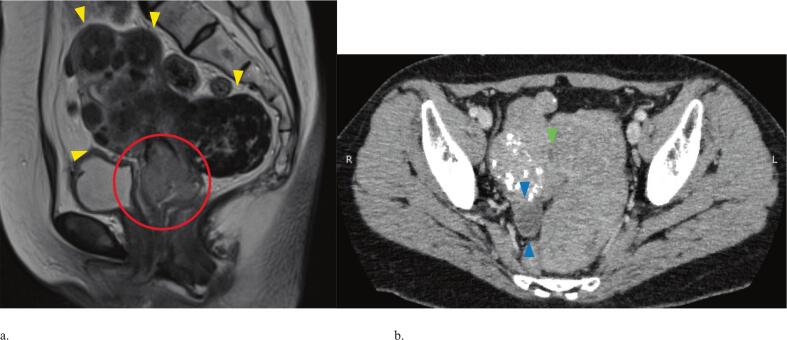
a. Pelvic magnetic resonance image at first visit showed multiple uterine fibroids, with the largest 8 cm in diameter (yellow arrowheads), and a cervical mass with parametrial infiltration (red circle). b. Computed tomography at first visit showed a 2 cm right hematosalpinx (blue arrowheads), no pyometra (green arrowhead). (For interpretation of the references to colour in this figure legend, the reader is referred to the web version of this article.)
